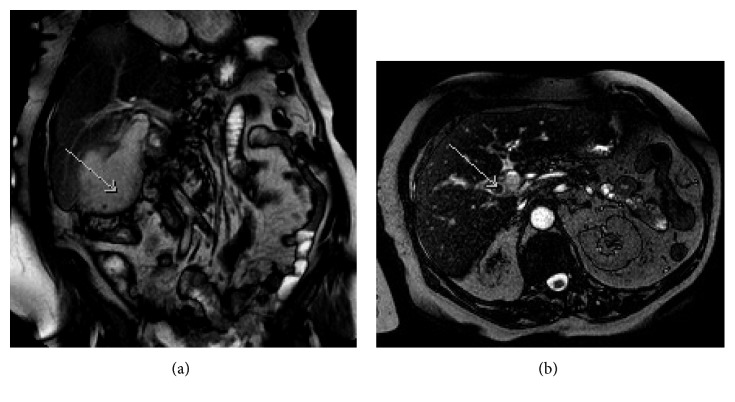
Coronal MRI image demonstrating large gallbladder empyema (a), and transverse MRI image section demonstrating 12 mm obstructing gallstone in the cystic duct (b).
